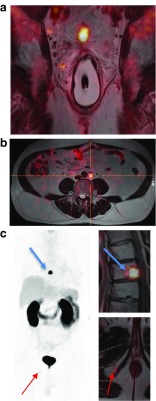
^68^Ga-PSMA-HBED-CC/^18^F-fluorocholine PET/MRI in prostate cancer: a locoregional lymph nodes; b paraaortic lymph node; c bone metastasis
